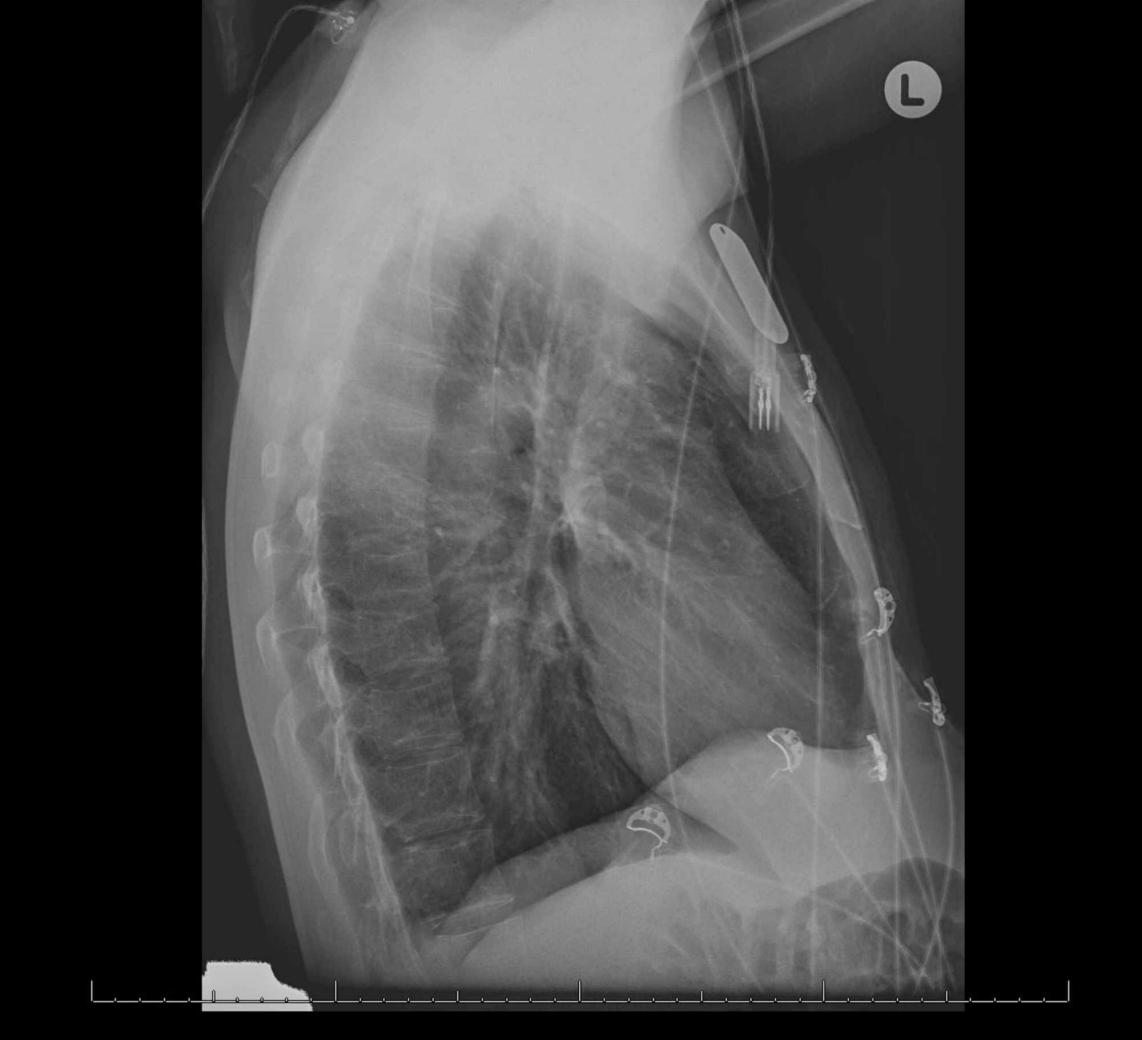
An unremarkable lateral chest radiograph on admission
